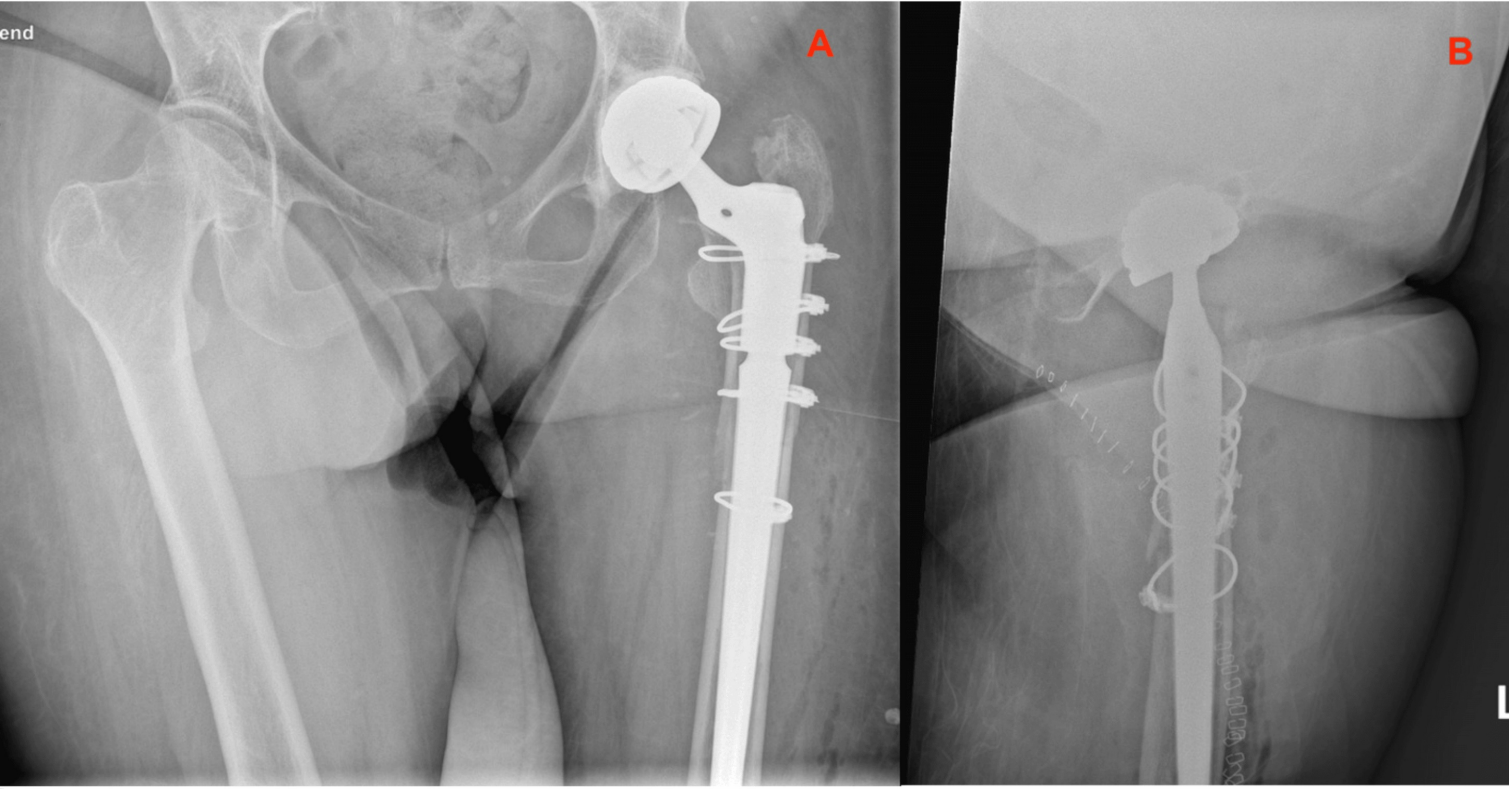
Total Hip Arthroplasty with Arcos stem and cerclages, direct after revision Routine direct postoperative radiographs of the revision Total Hip Arthroplasty with Arcos femoral stem and cerclages (Zimmer Biomet, Warsaw, USA), performed for a Vancouver B2 periprosthetic hip fracture. 5A: Anterior Posterior view of the Pelvis; 5B: Lateral view of the Left Hip
